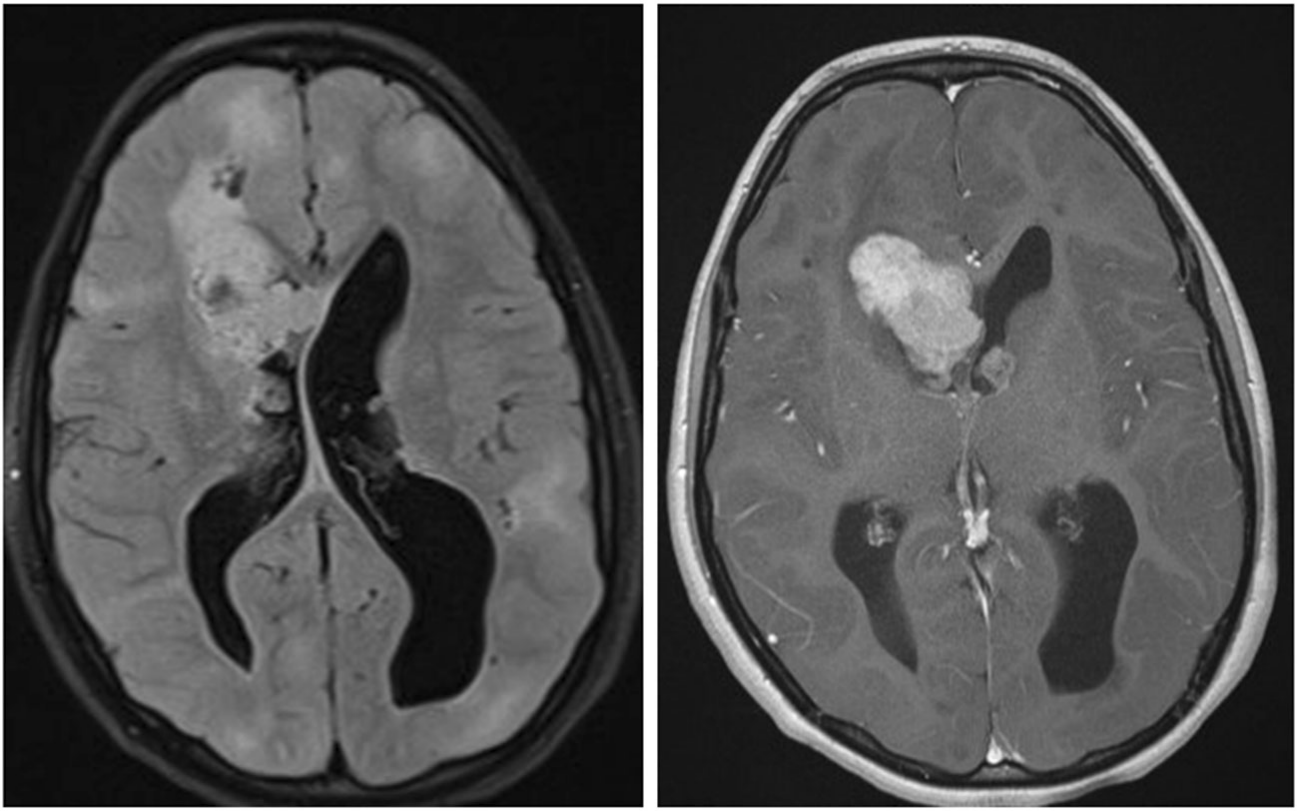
Subependymal giant cell astrocytoma (SEGA): axial FLAIR and T1-contrast-enhanced MRI of a 12-year-old girl with known tuberous sclerosis and epilepsy, presenting with new-onset symptoms of increased intracranial pressure. MRI shows multiple cortical tubers, and bilateral SEGA (prominent on the right side) with obstructive hydrocephalus. The child underwent transcortical resection of the tumor and a septostomy. No progression of the left tumor or recurrence of the right tumor is evident over a 6-year follow-up
